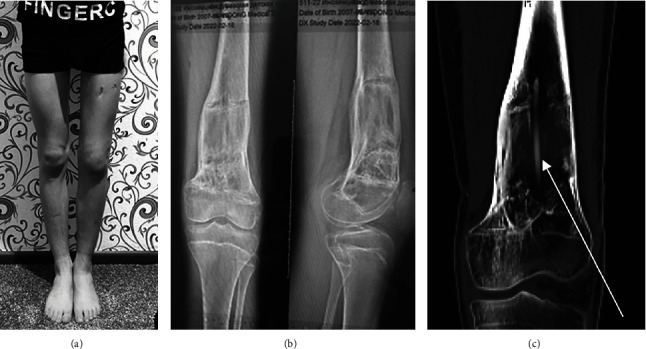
After frame removal: (a) photo; (b) AP and lateral view radiographs (2 months after frame removal); (c) CT longitudinal scan at 6-month follow-up with a PCL/HA implant (arrow) visible in newly formed bone.
